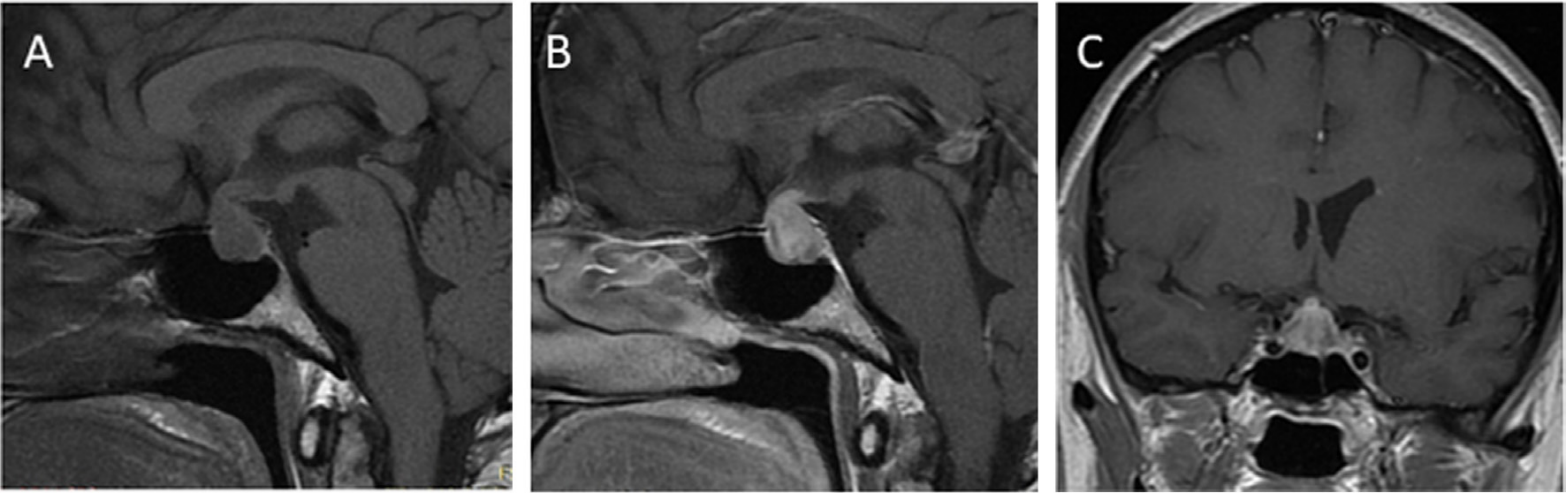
Pituitary MRI: sagittal T1-weighted images before (A) and after gadolinium enhancement (B) and a coronal T1-weighted image after gadolinium enhancement (C) in a 56-year-old female patient with melanoma who developed ICI-induced hypophysitis after the third dose of ipilimumab.
